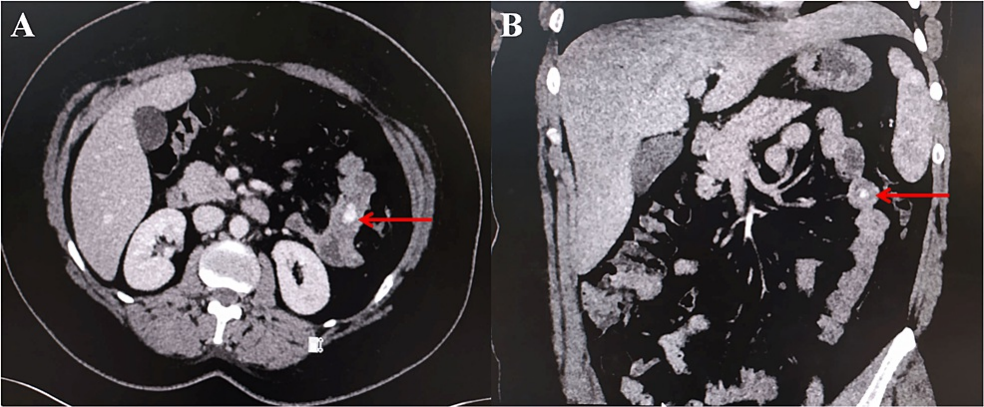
Contrast-enhanced computed tomography shows intraluminal extravasation of the intravenous contrast in the jejunum (red arrow) on axial and coronal sections (A, B).
